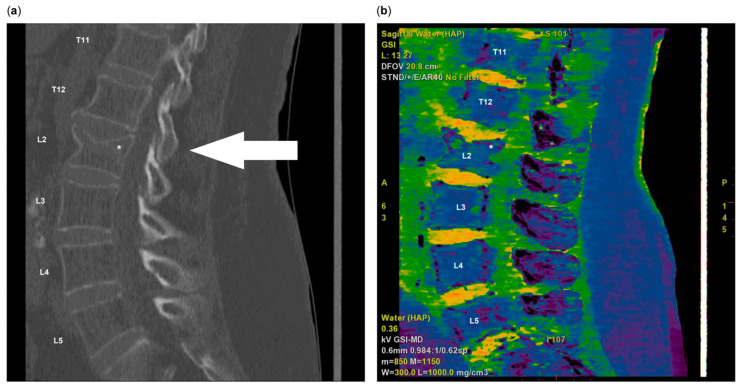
Computed tomography (a) and the corresponding dual-energy image (b) of a previously unknown L2 vertebral fracture in a 79-year-old female patient. The presence of yellow colouring is indicative of increased fluid content, which is characteristic of the ligamentous disc or oedema observed in acute fractures. Conversely, the homogeneous blue colouring of the vertebral body is an indication that the fracture is a old one. The arrow denotes the vertebral body that has been affected, whilst the asterisk indicates the location of the endplate fracture.
